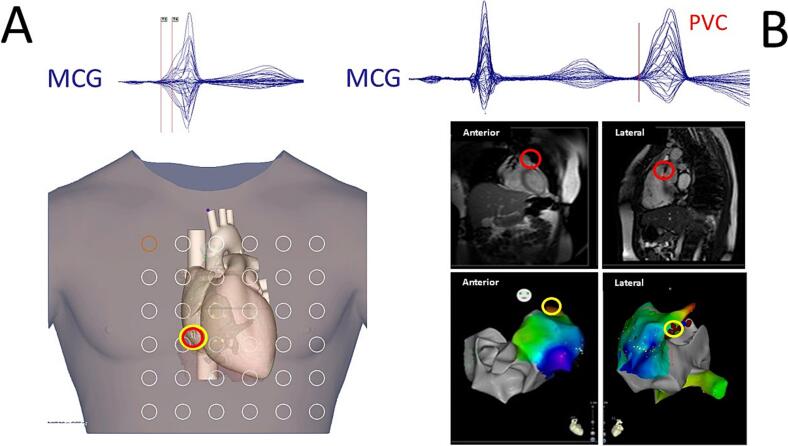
Examples of successfully ablated MCG localized arrhythmogenic substrates: (A) Right lateral accessory pathway (multimodal MCG-CT-Carto integration). (B) Right ventricular outflow tract (RVOT) bigeminy (multimodal MCG-MRI-Carto integration). The sites of MCG localization and successful ablation are highlighted with red and yellow circles respectively. MCG: “Butterfly” overlays of the 36-channel waveforms). (For interpretation of the references to colour in this figure legend, the reader is referred to the web version of this article.)
